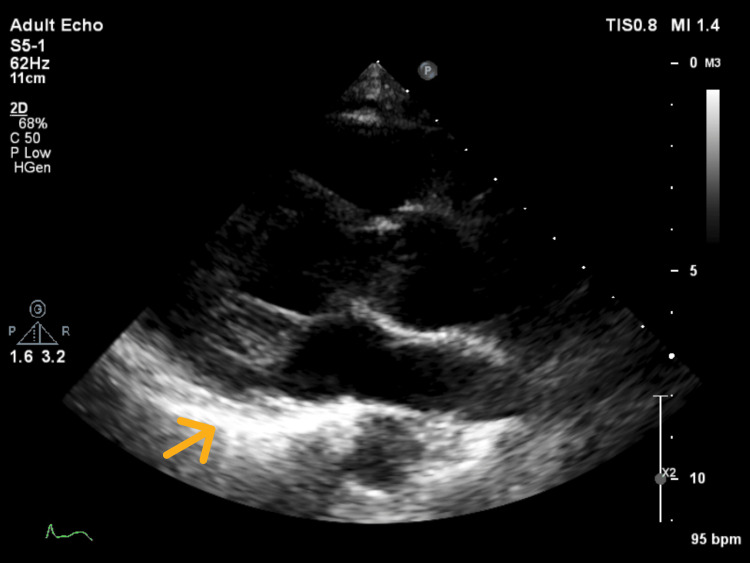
Two-dimensional echocardiogram The arrow indicates a thickened and shinning pericardium.
